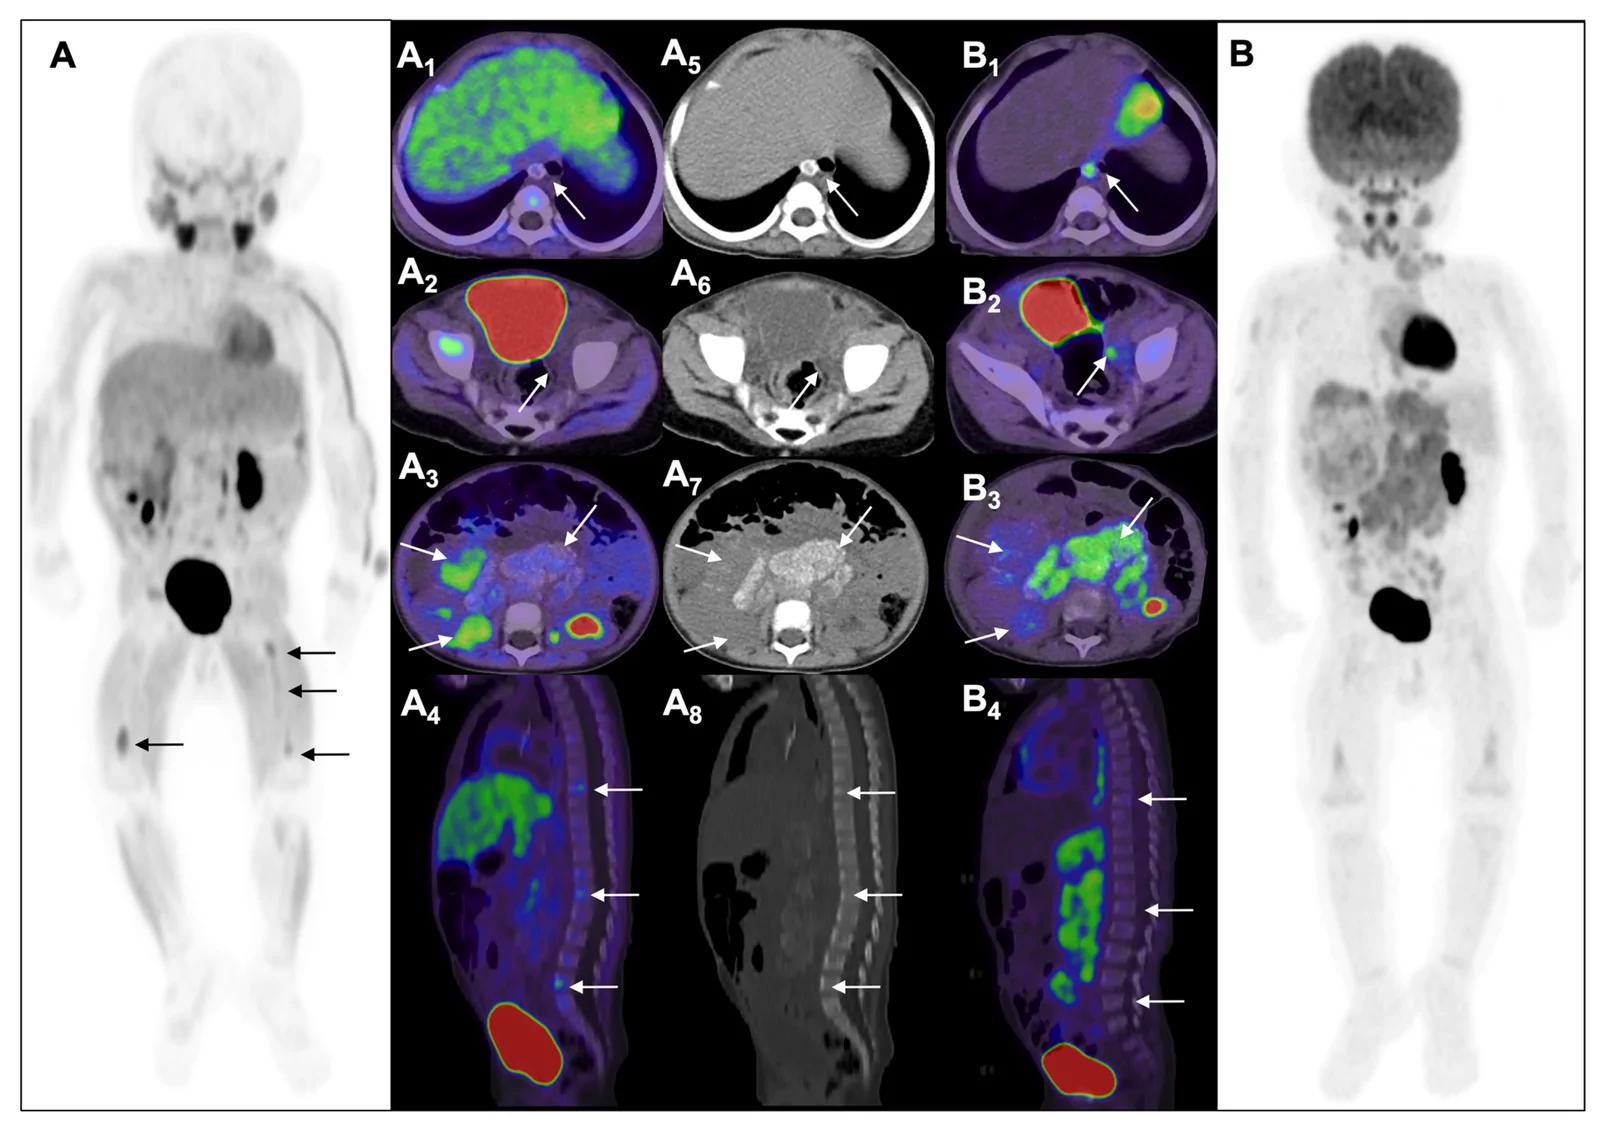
Divergent functional phenotypes between NET-targeted [^18^F]MFBG PET and [^18^F]FDG PET in an 11-month-old boy with newly diagnosed neuroblastoma. [^18^F]MFBG PET image (A) demonstrating diffuse bone marrow involvement in both lower extremities (arrows). Axial PET/CT fusion images show no MFBG uptake in enlarged calcified mediastinal (A_1_) and pelvic lymph nodes (A_2_). The retroperitoneal primary tumor (A_3_) demonstrates heterogeneous MFBG uptake, with relatively lower uptake in calcified components and higher uptake in soft-tissue portions. Sagittal fusion image (A_4_) demonstrates multiple focal MFBG-avid lesions. Corresponding CT images (A_5_–A_8_) show calcified lymph nodes and primary tumor without osseous destruction. In contrast, [^18^F]FDG PET MIP (B) and fusion images demonstrate intense uptake in lymph nodes (B_1_, B_2_), heterogeneous uptake in primary tumor (B_3_), however, no focal uptake on spine (B_4_). The lower extremities’ uptake appeared to be symmetric, making the distinction between marrow metastases and reactive changes uncertain. Following four cycles of induction chemotherapy, the patient underwent radical tumor resection with systematic lymph node dissection. Pathology confirmed metastatic neuroblastoma in both the calcified mediastinal and pelvic lymph nodes.
